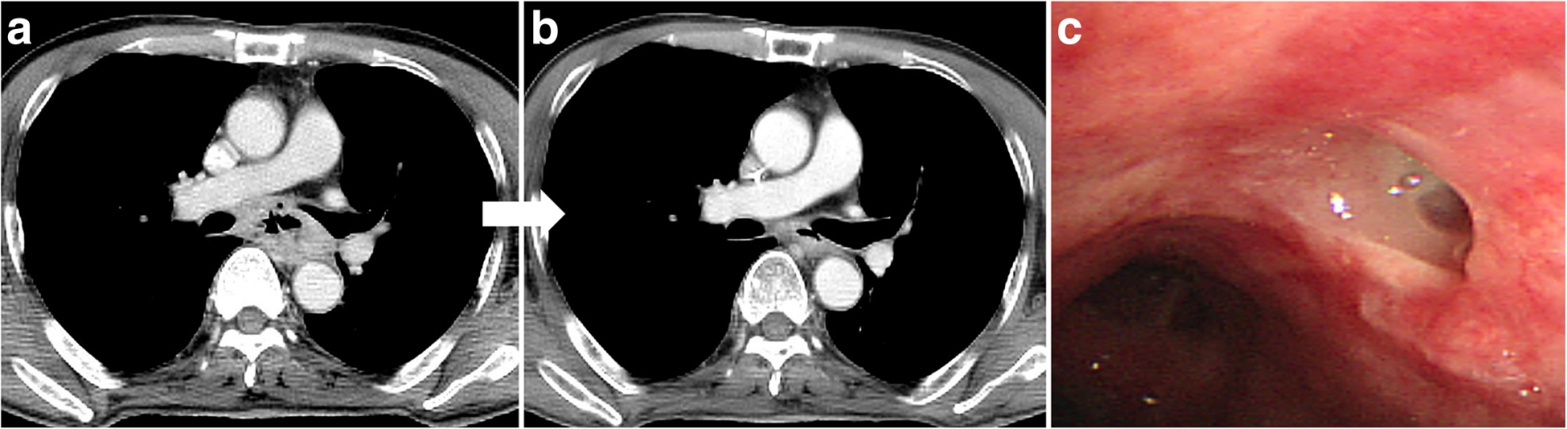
Chest CT and bronchoscopy images at pre-treatment (a) and 1-month follow-up (b and c) in a patient with newly developed bronchoesophageal fistula after treatment
